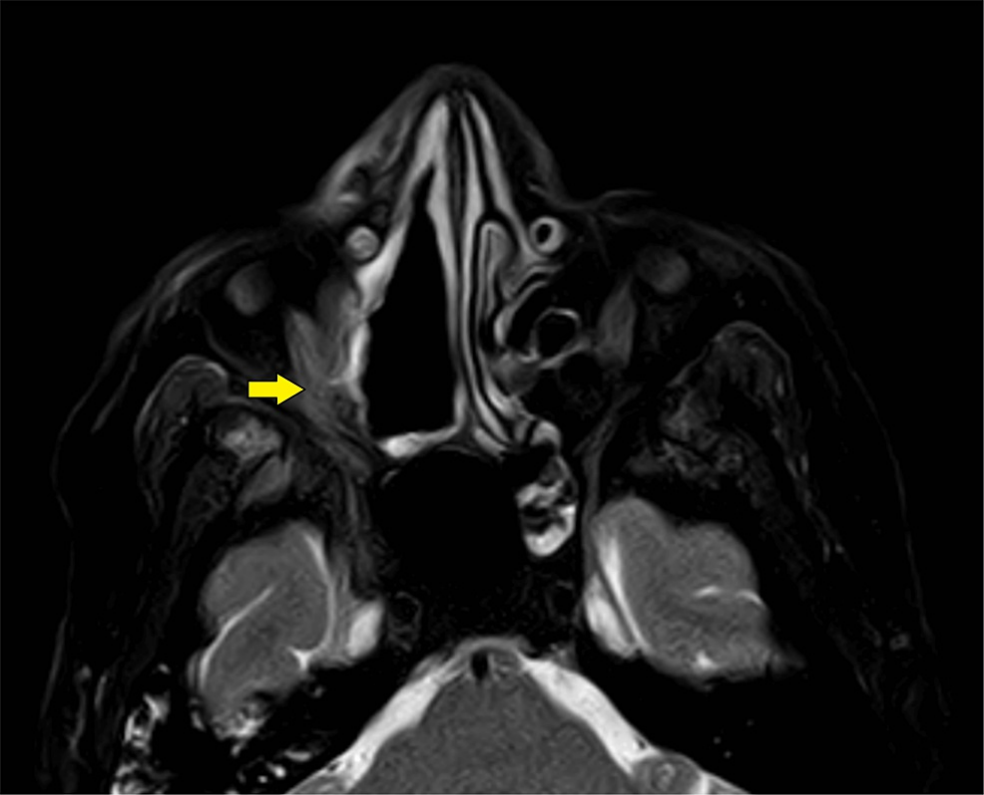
Magnetic resonance imaging (T2 fat-saturated axial section) showing obliteration of the retro-antral fat on the right side with high signals in the surrounding tissues (arrow) suggestive of disease extension into the infratemporal fossa.
